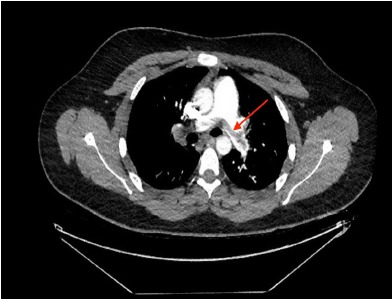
Axial view of the chest on computed tomography for pulmonary angiogram, demonstrating a saddle pulmonary embolism (arrow), with near-occlusive clot in the right pulmonary artery.
